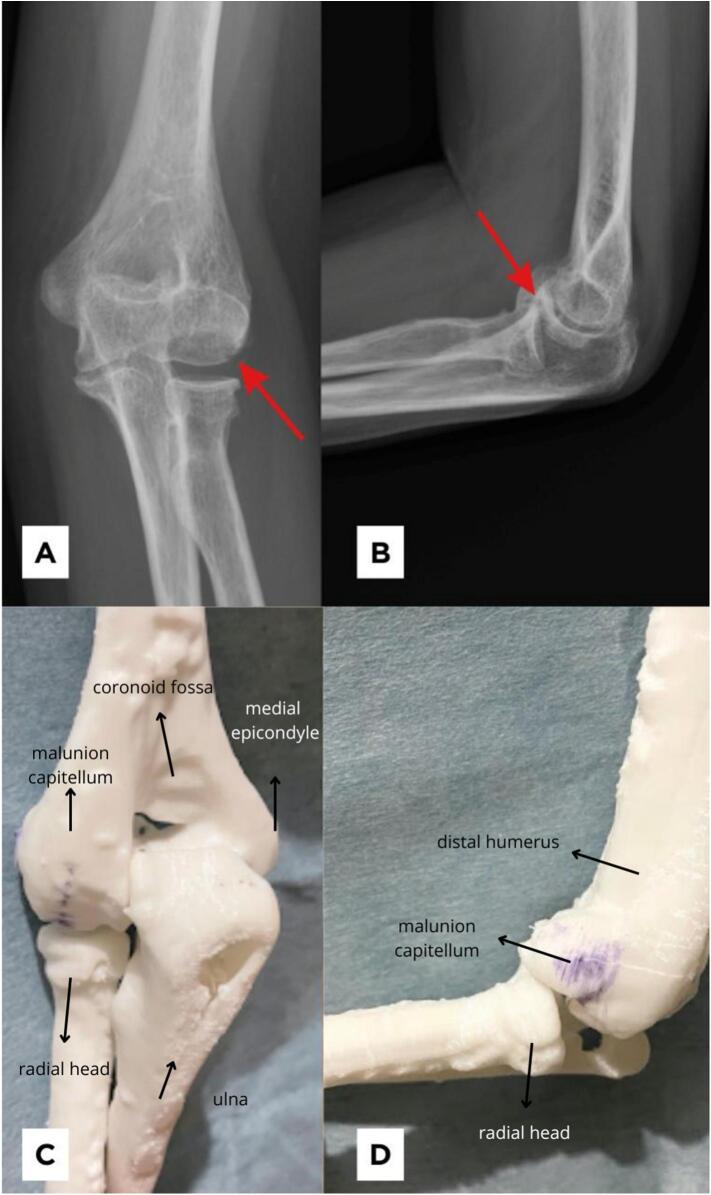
Imaging studies of left elbow. Plain radiograph of the elbow in (A) AP position and (B) lateral position, showing the characteristic double-arc sign (arrow) revealing a malunited (coronal sheer) in humeral capitellum. (C&D) A 3D-printed model of the patient's left elbow was made based on a CT scan showing a malunion of humeral capitellum.
